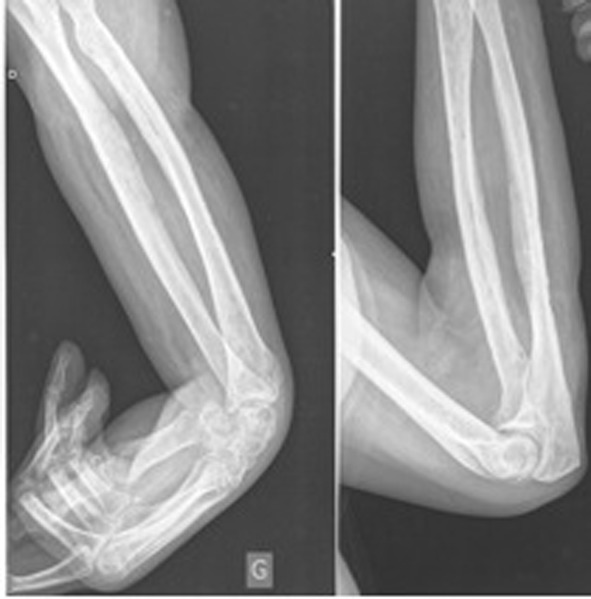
X-rays performed 12 months after cordarone extravasation show a severe contracture of the upper limb involving elbow, wrist and interphalangeal joints of the fingers. Notice the atrophy of the soft tissues of the forearm (reduced diameter of the forearm and hypo-density due to the fatty-fibrous atrophy).
